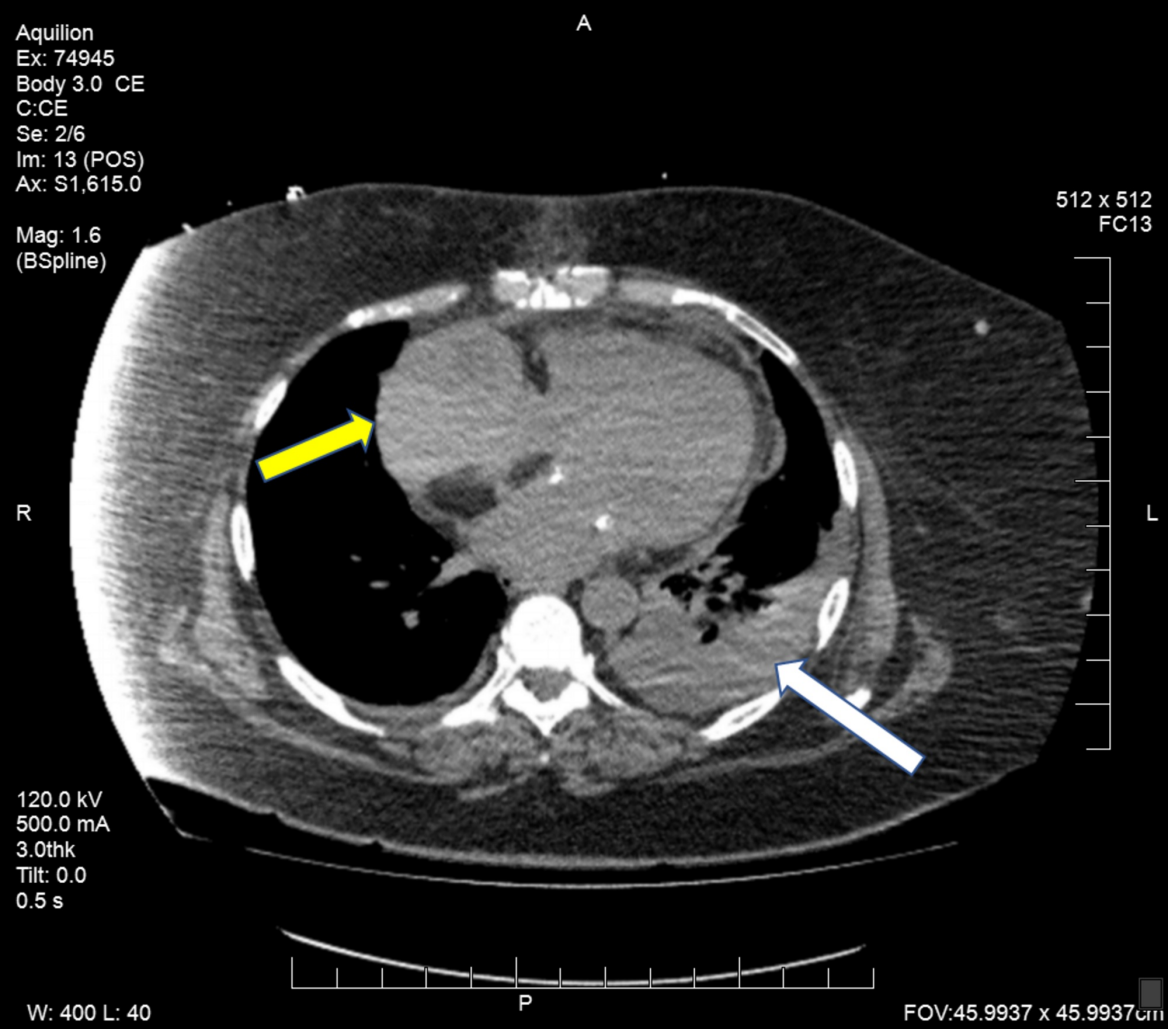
CT scan of the chest showing pericardial hematoma Computed tomography (CT) scan of the chest showing a pericardial hematoma indenting the right atrium (yellow arrow) and left lower lobe pulmonary infiltrate (white arrow)
